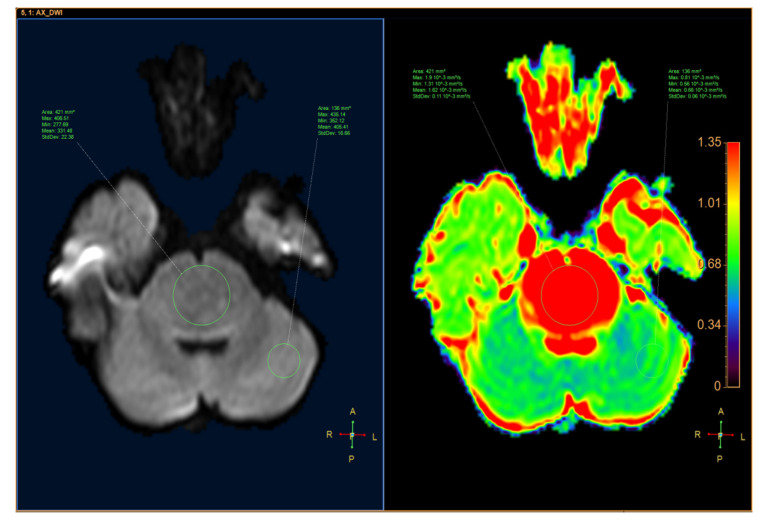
A 10-year-old male patient had a tumor located in the pons, which was diagnosed as a diffuse glioma. (Left) Axial DWI image. (Right) ADC map.
